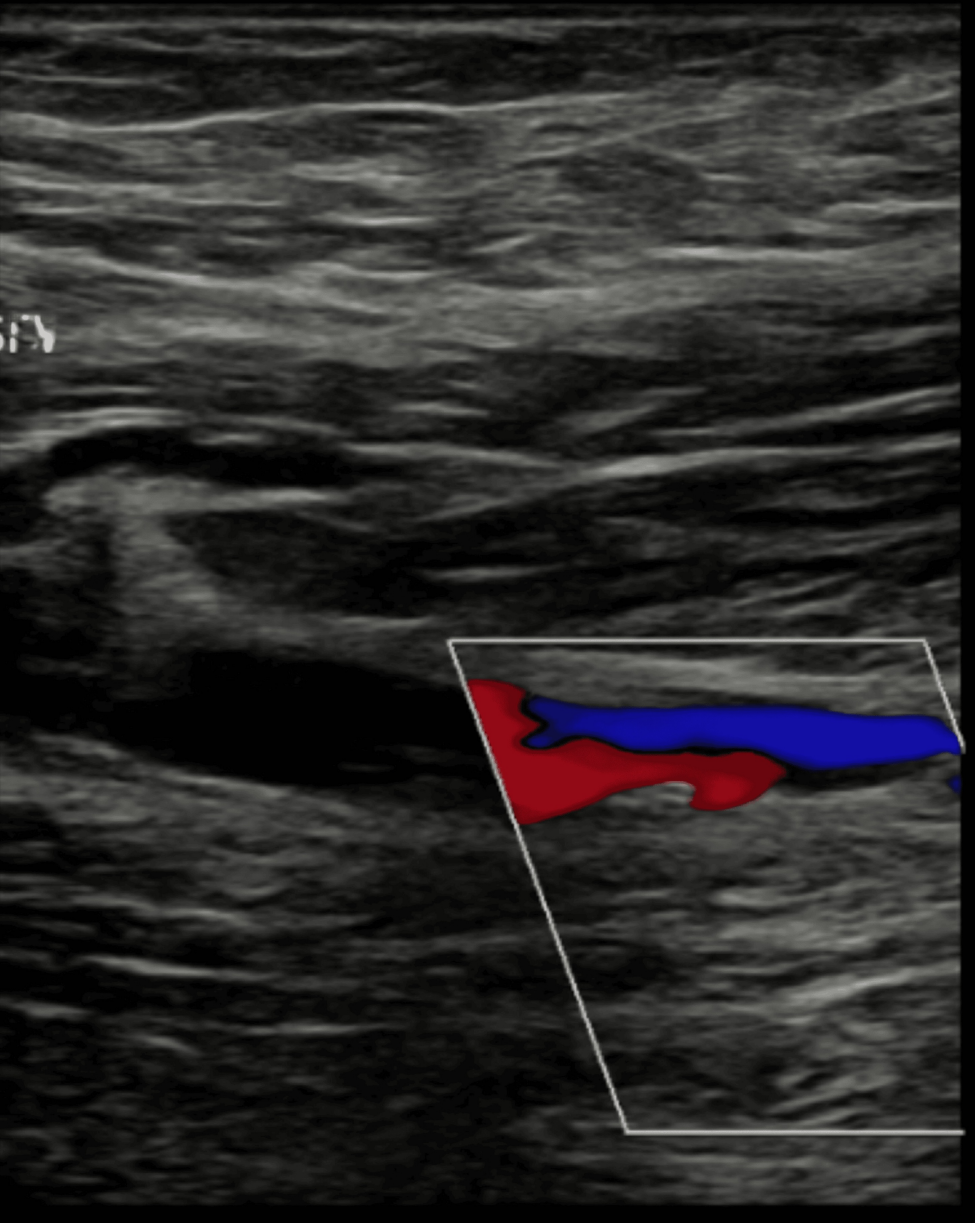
Shows another USS image of the left distal superficial femoral vein where a chronic DVT was visualized. USS: ultrasonography scan; DVT: deep venous thrombosis
